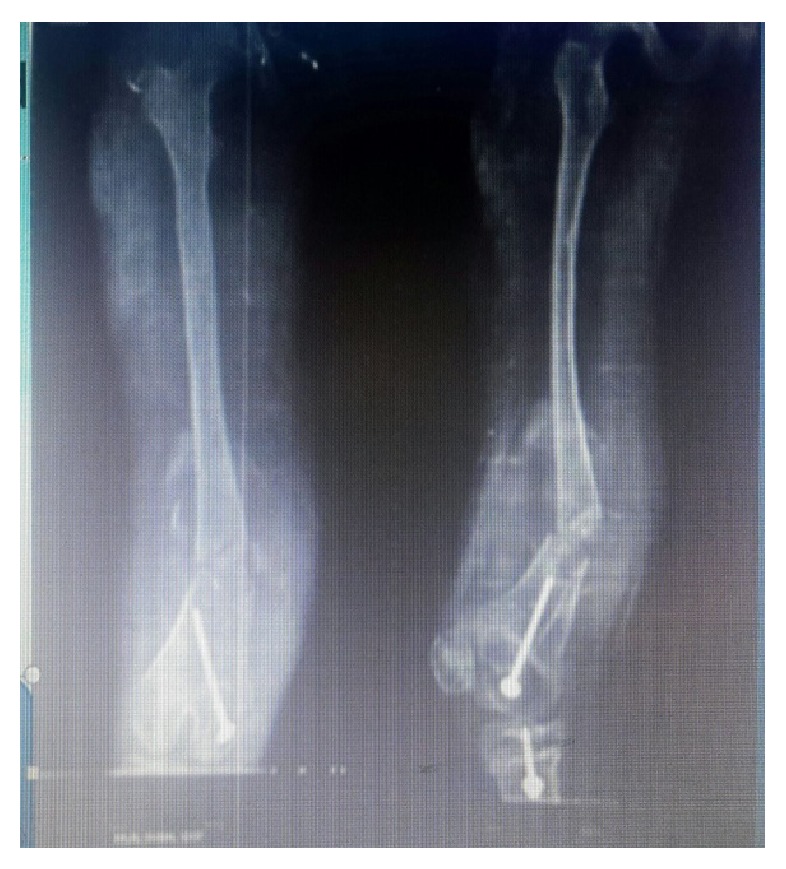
Plain X-ray of right thigh anteroposterior and lateral views showing fracture of femur at junction of middle and distal third with screws in situ used for hemiepiphysiodesis.
